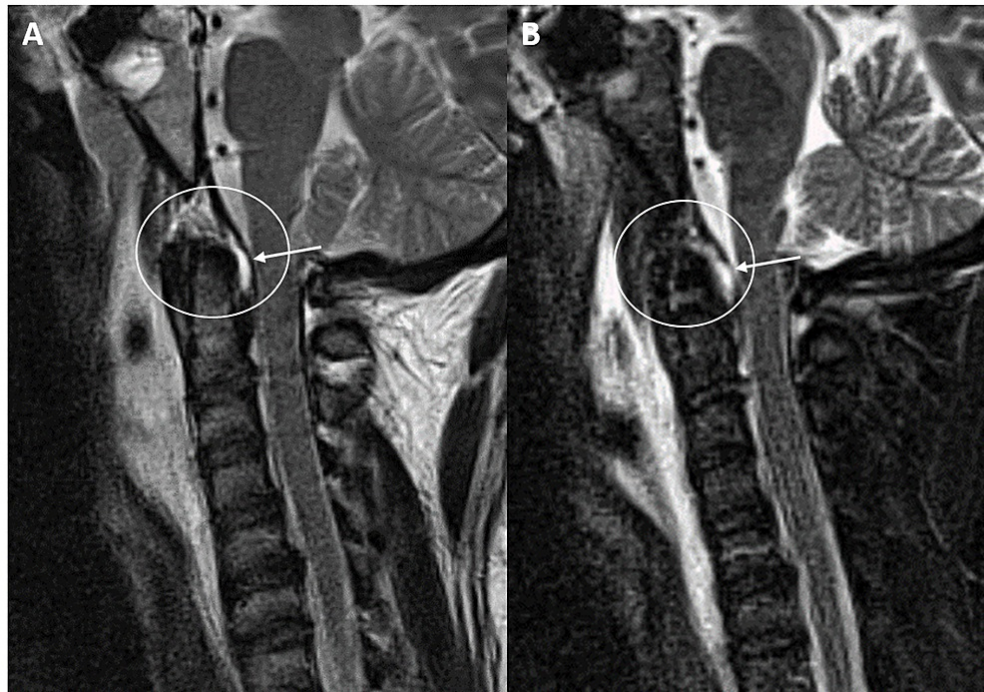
A and B: Sagittal T2 and STIR MRI sequences of the craniocervical junction and cervical spine demonstrate a subclival tectorial membrane tear posterior to the dens (white arrows/circles) near the ligamentous insertion in a 71-year-old female status post motor vehicle collision. Additional tears of the anterior longitudinal ligament at C1 and posterior atlantooccipital membrane complex are demonstrated. STIR = short tau inversion recovery
